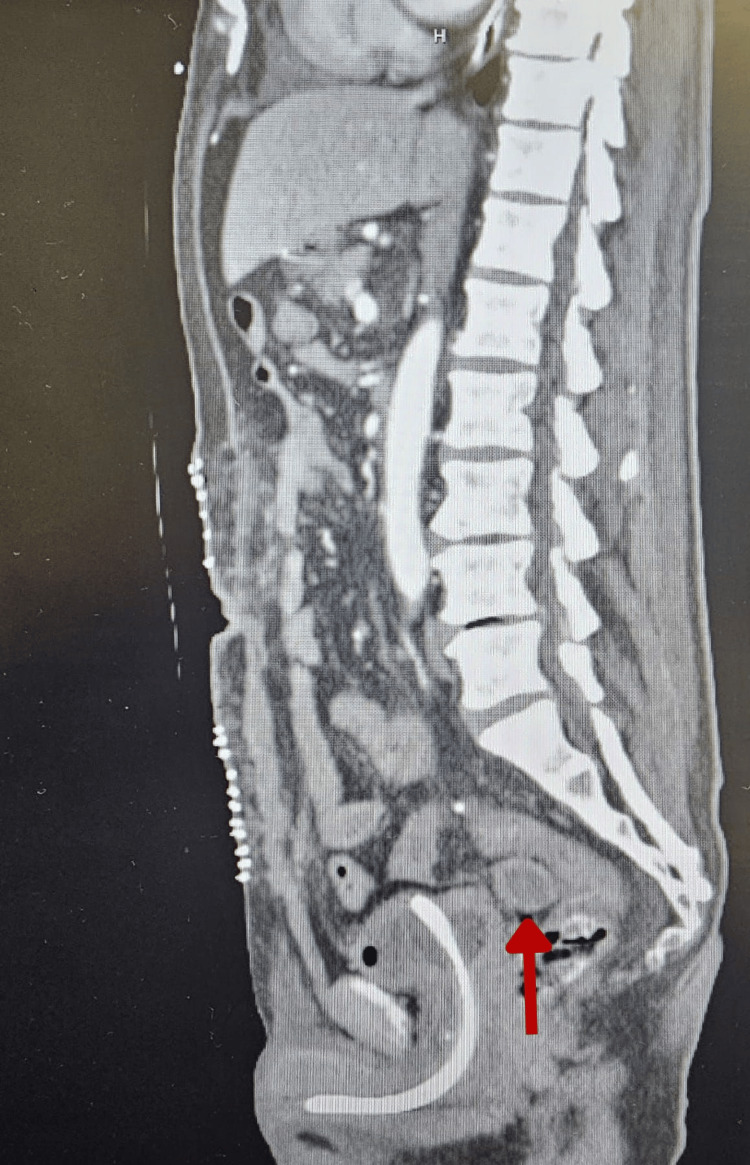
CT angiogram of the abdomen and pelvis describing a 3.5 cm fluid collection between the bladder and rectum (arrow).
